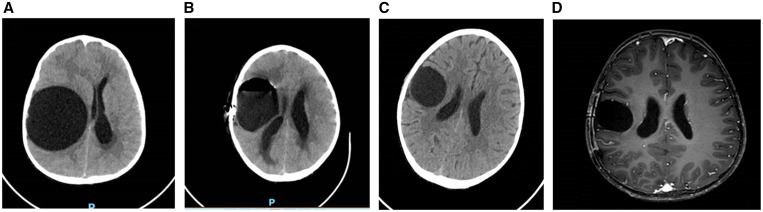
(A–C) Brain CT – axial view without contrast: (A) brain cyst with daughter cyst – pre surgery; (B) day 1, post-surgery; (C) 2 months post-surgery. (D) Brain MRI – axial T2 view without contrast, 4 months post-surgery.
